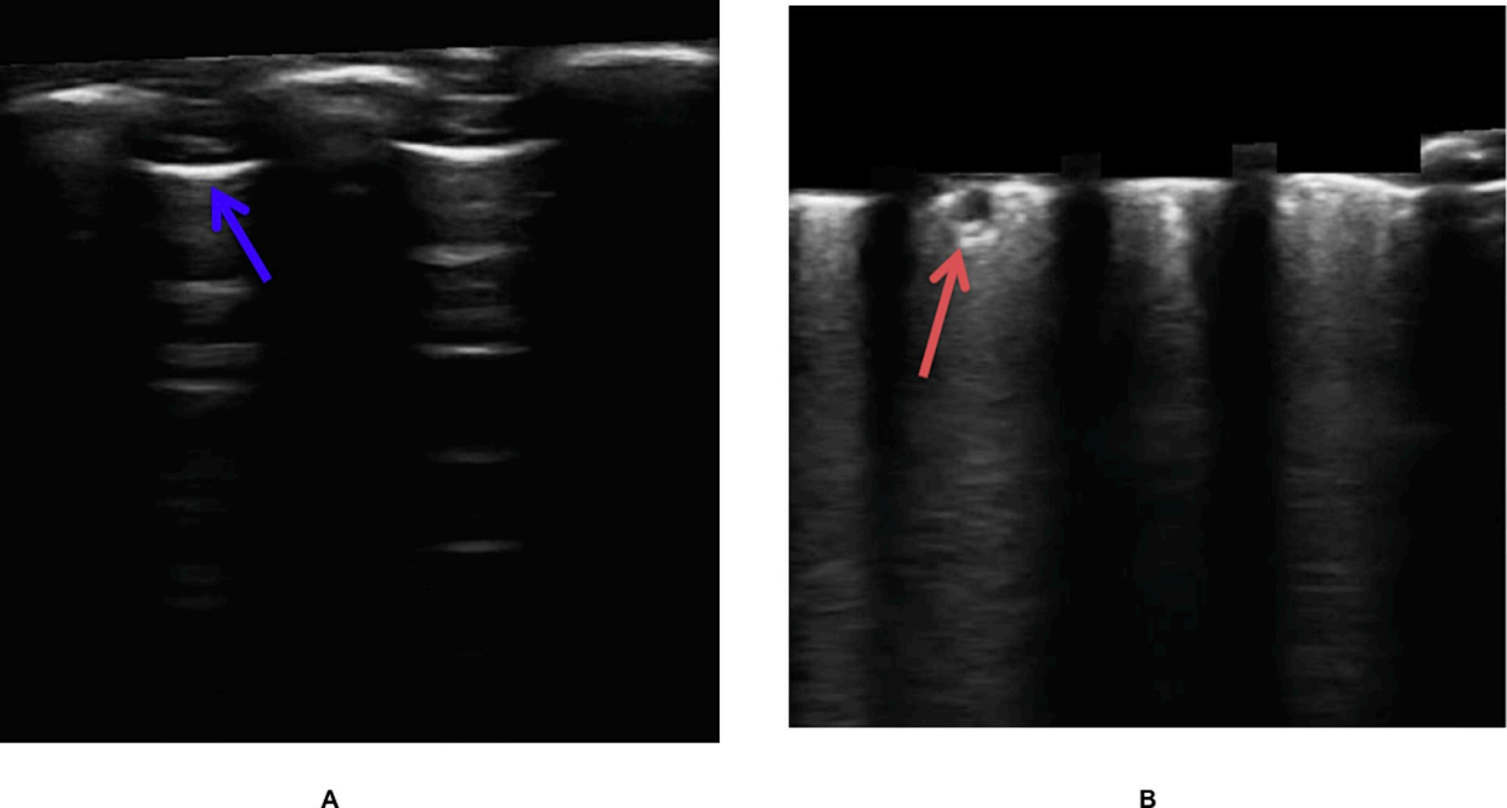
Automatic identification of the pleural line by the algorithm and removal of the skin. (A) ultrasound image of a healthy lung where the pleura is identified between two rib-bones (blue arrow), (B) ultrasound image of the lung where the soft tissues were removed and with evidence of infiltrate (red arrow).
